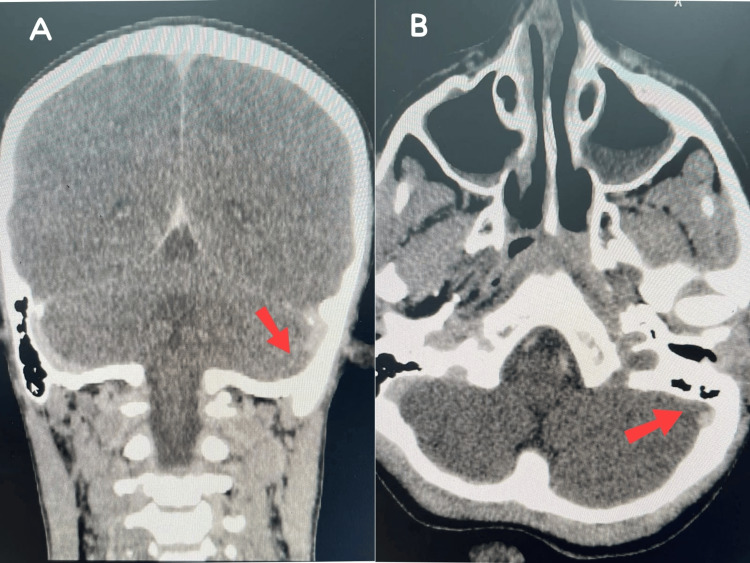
Head CT scan with IV contrast showing a filling defect within the left internal jugular vein extending to the ipsilateral sigmoid sinuses (A-B). (A) Section indicating sigmoid sinus thrombosis; (B) Section showing left internal jugular vein thrombosis.
